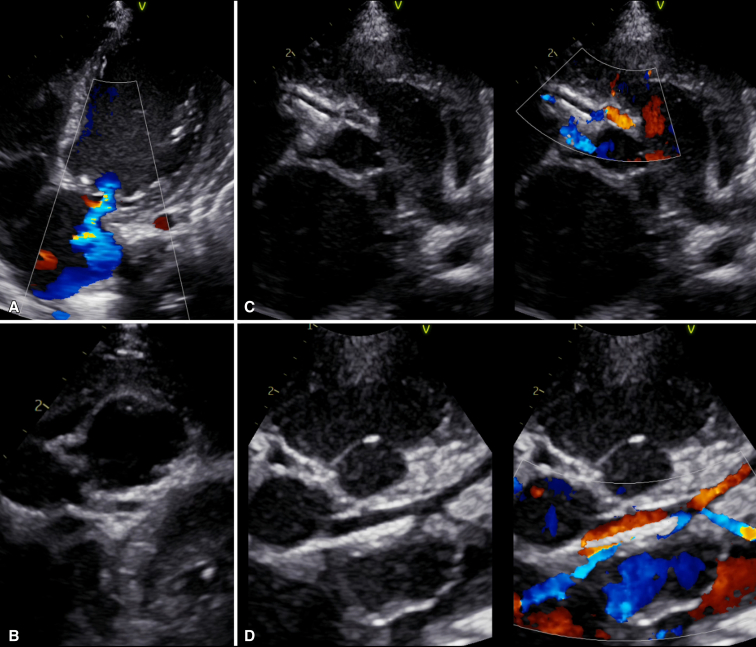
Echocardiographic views demonstrating coronary and valvular anomalies. A, Parasternal 4-chamber view showing ischemic mitral regurgitation. B, Short-axis view falsely suggesting a normal origin of the left coronary artery from the left coronary sinus. C, Short-axis view demonstrating the right coronary artery arising from the pulmonary artery. D, High short-axis view confirming the anomalous origin of both the right and left coronary arteries from the pulmonary artery trunk, with evidence of an intramural course. Unlike anomalous left coronary artery from the pulmonary artery, the left coronary artery flow was observed in red and no collateral vessels or right coronary artery dilatation were identified.
